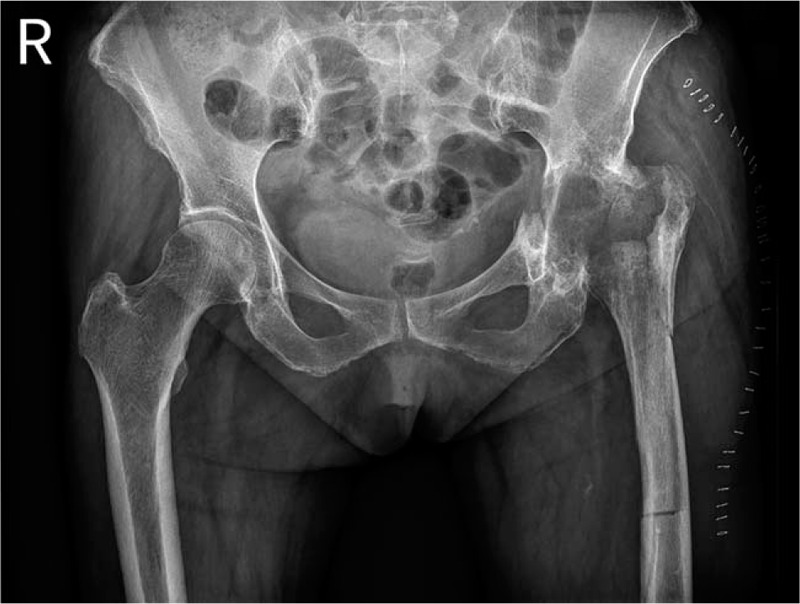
X-ray showed changes after removal of hip prosthesis.
